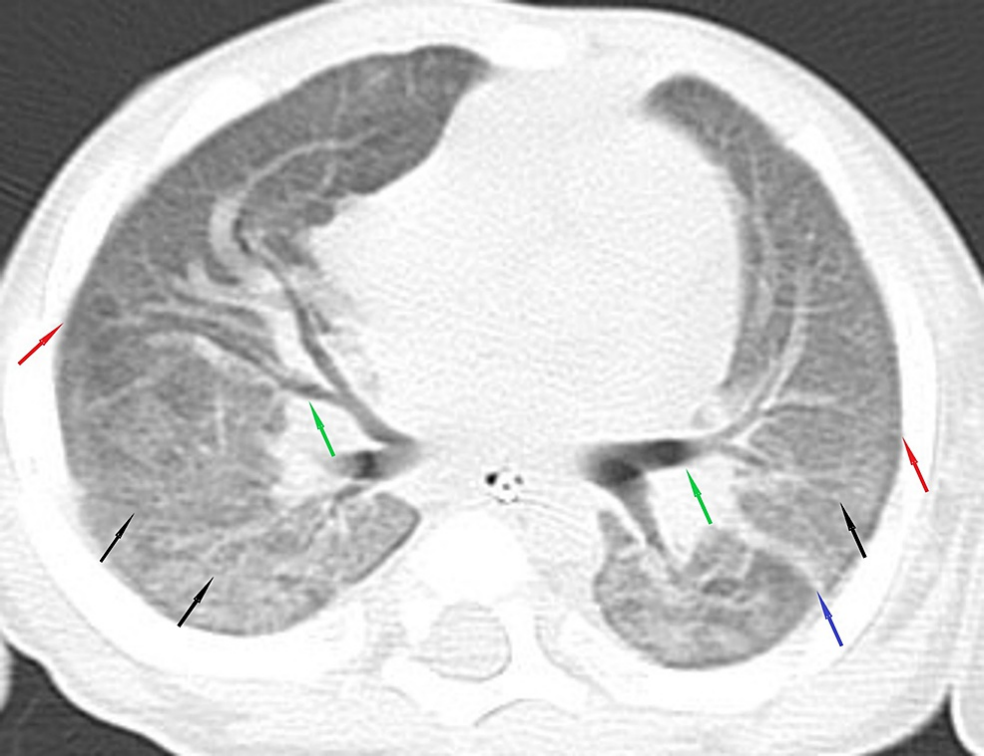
High-resolution chest CT scan. High-resolution chest CT scan revealed diffuse ground-glass opacities (black arrows), septations (blue arrow), central bronchiectasis (green arrows), subpleural small cysts (red arrows), and fibrotic changes.
